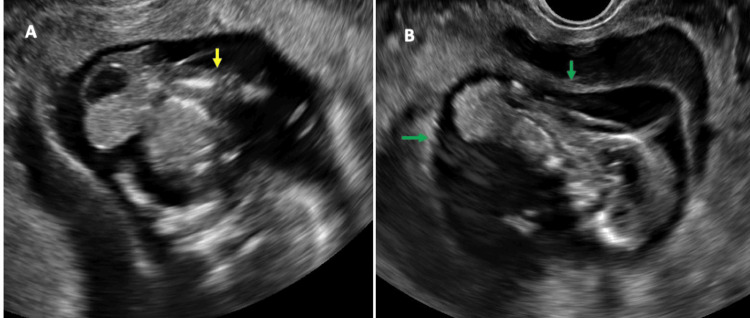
USG image showing eviscerated organs with a deformed left lower limb (yellow arrow) (A), and a wide chorioamniotic separation (green arrow) (B). USG, ultrasound
